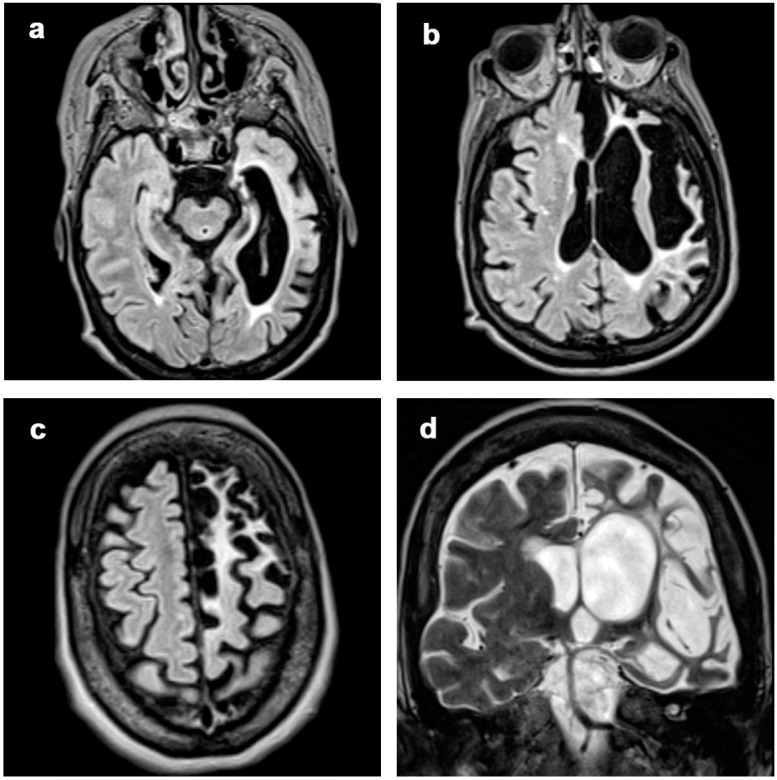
Patient 13, with stable atrophy changes during 12 years of MRI controls. Axial FLAIR images (a–c) and coronal T2-weighted image (d) at a single timepoint, showing frontal, insular and temporal atrophy with SI involving subcortical WM and cortical GM. The patient has a 25 years of disease duration with clinical onset with focal deficits, seizures and cognitive disorders; at last follow-up, EPC and behavioral disorders are present.
